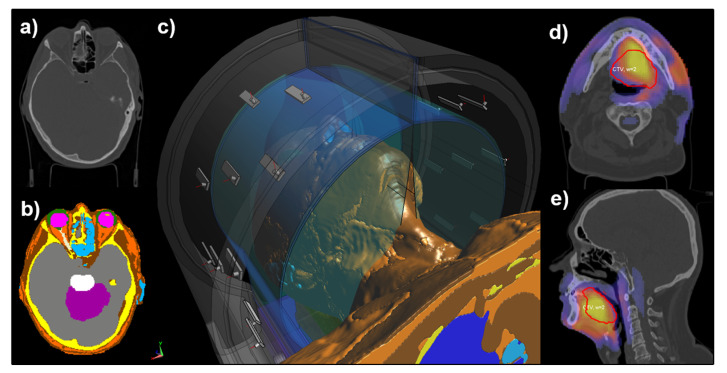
Overview of the Erasmus MC procedure of hyperthermia treatment planning for H&N hyperthermia with the HYPERcollar3D applicator. (a) example of axial CT slice, (b) corresponding segmentation in iSeg, (c) hyperthermia treatment planning setup in SEMCAD X, (d) axial and (e) sagittal slices of SAR with highlighted target region.
